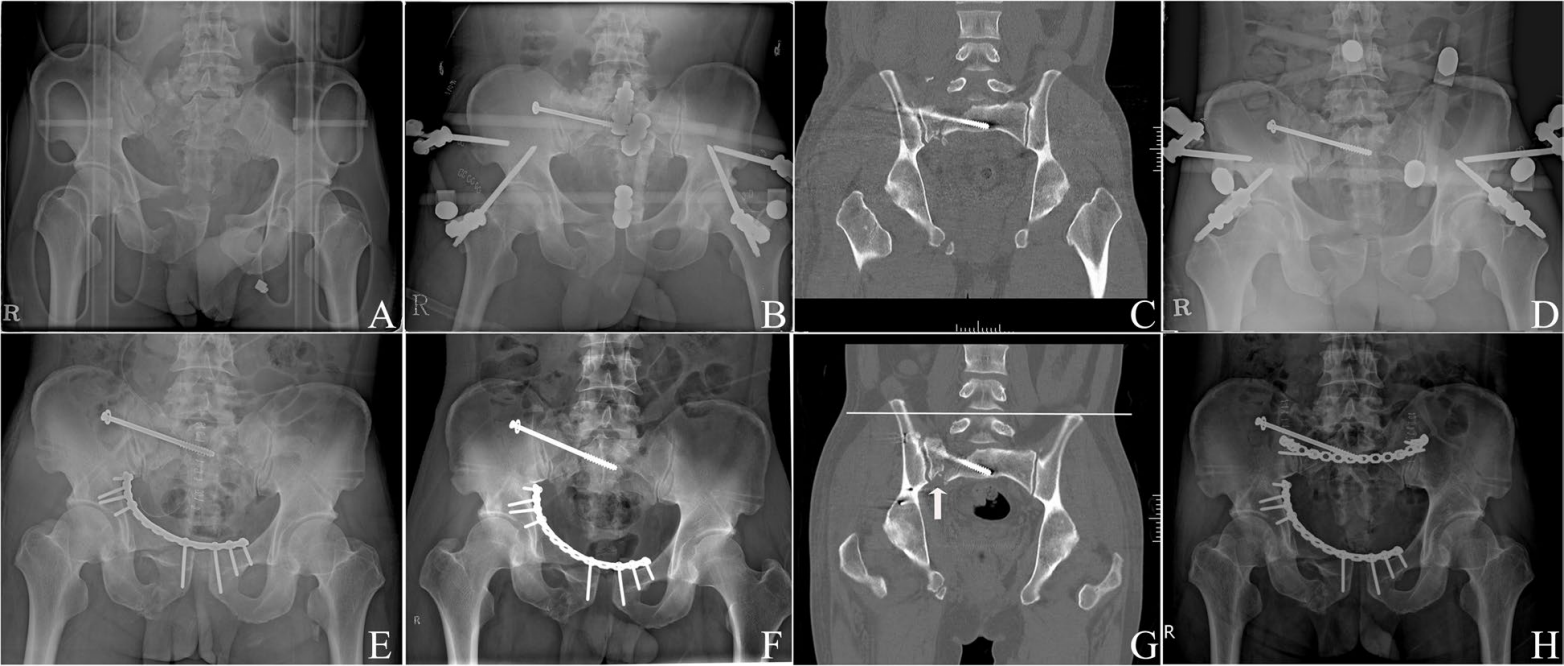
A A 30-year-old man with a Tile C-type pelvic ring injury. B External fixation and iliosacral (IS) screw fixation on the day of injury. C Coronal reconstructed image showing a well-reduced posterior ring. D Post-injury radiograph at 4 weeks showing IS screw loosening. Definitive treatment was delayed due to unstable conditions of the patient with multiple trauma including aortic dissection. E Anterior plating 1-month post-injury. F Pelvic radiograph showing IS screw migration at 8 weeks post-injury. G Coronal image at 8 weeks post-injury showing vertically displaced sacral alar (arrow) and upward migration of right pelvis (white line). H Posterior transiliac plating was performed to acquire a more rigid fixation at 2 months post-injury
